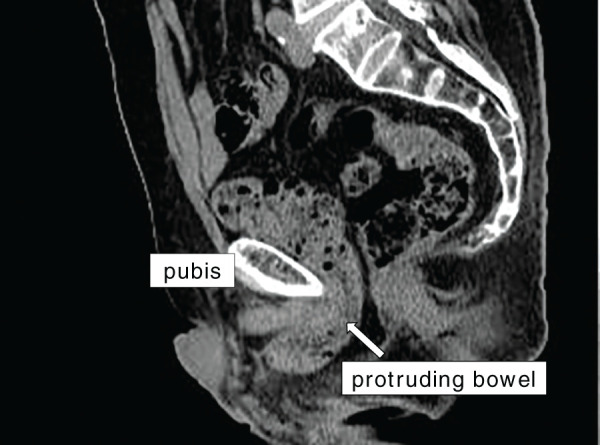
Preoperative CT scan of Case 1. Small bowel herniation is observed anterior to the superficial transverse perineal muscle, leading to the diagnosis of SAPH. SAPH, secondary anterior perineal hernia
